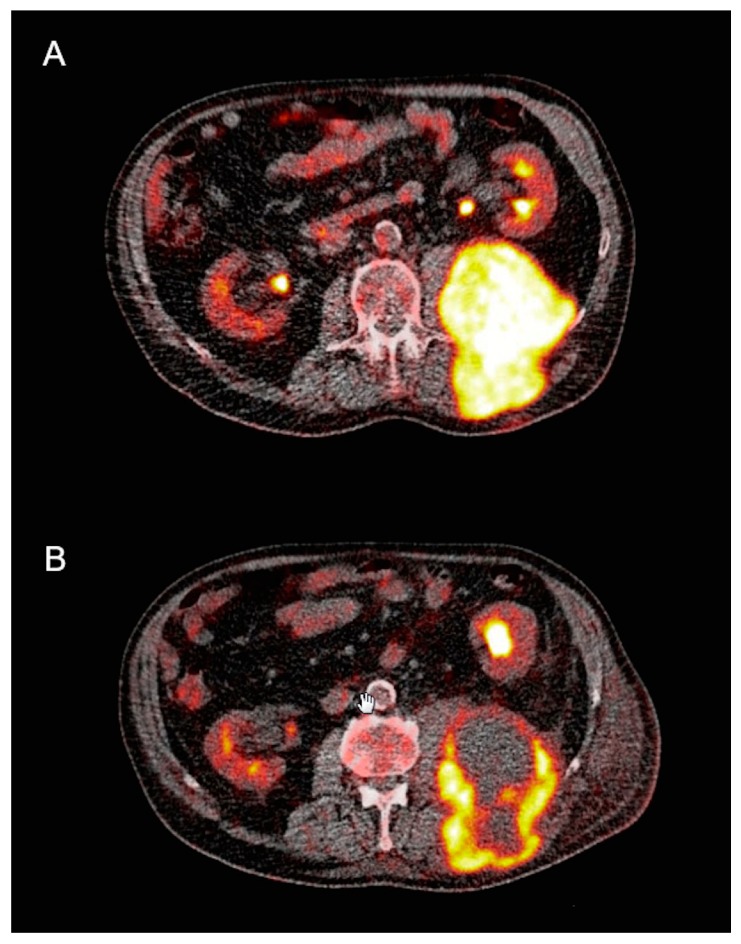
Transaxial fludeoxyglucose F-18 positron emission tomography/computed tomography (^18^F-FDG PET/CT) of an 80-year old male patient with retroperitoneal sarcoma infiltrating the back muscles before (A) and after pazopanib therapy (B). Clear metabolic remission of the initially intense metabolic lesion with areas of central necrosis in response to pazopanib.
